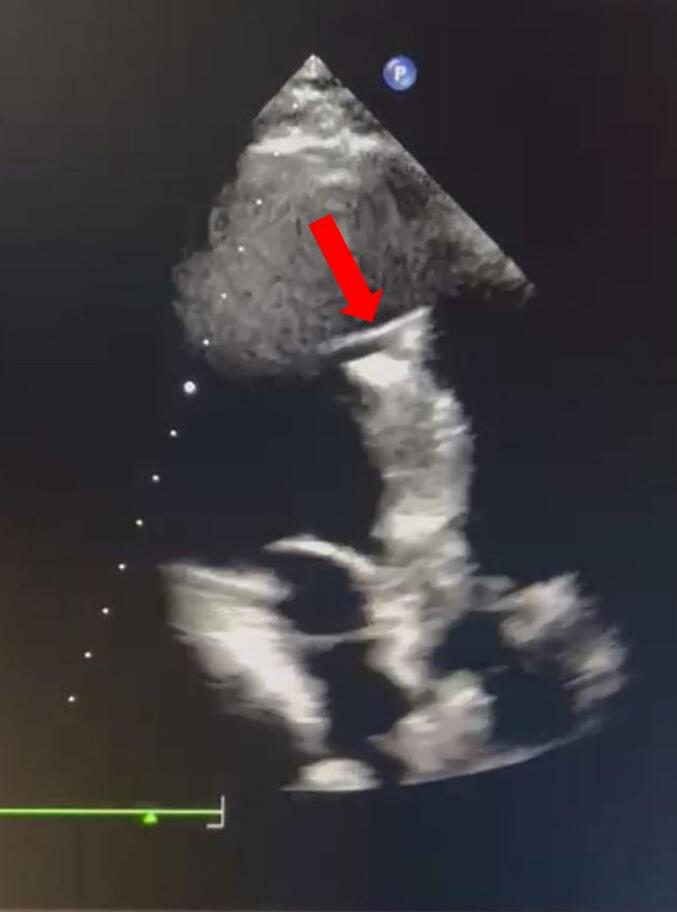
Apical three-chamber view of the 2D transthoracic echocardiogram. The red arrow shows the metallic shadow of the needle in the LV.
